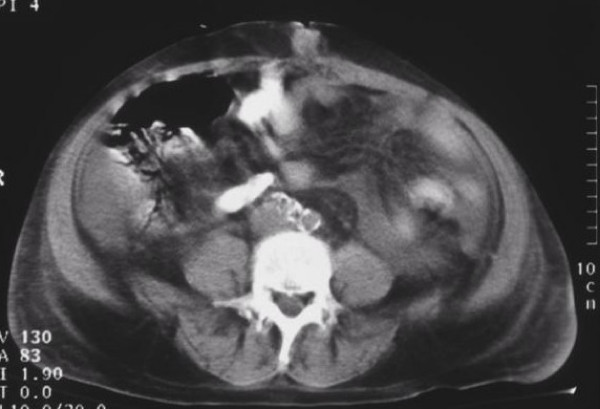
CT-scan of the abdomen: This image shows an umbilical mass measured 2.5 cm, involving the adjacent adipose tissue.
